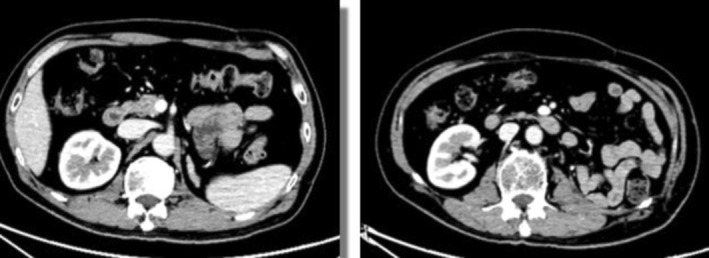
Urinary CT was reexamined in March 2018.
